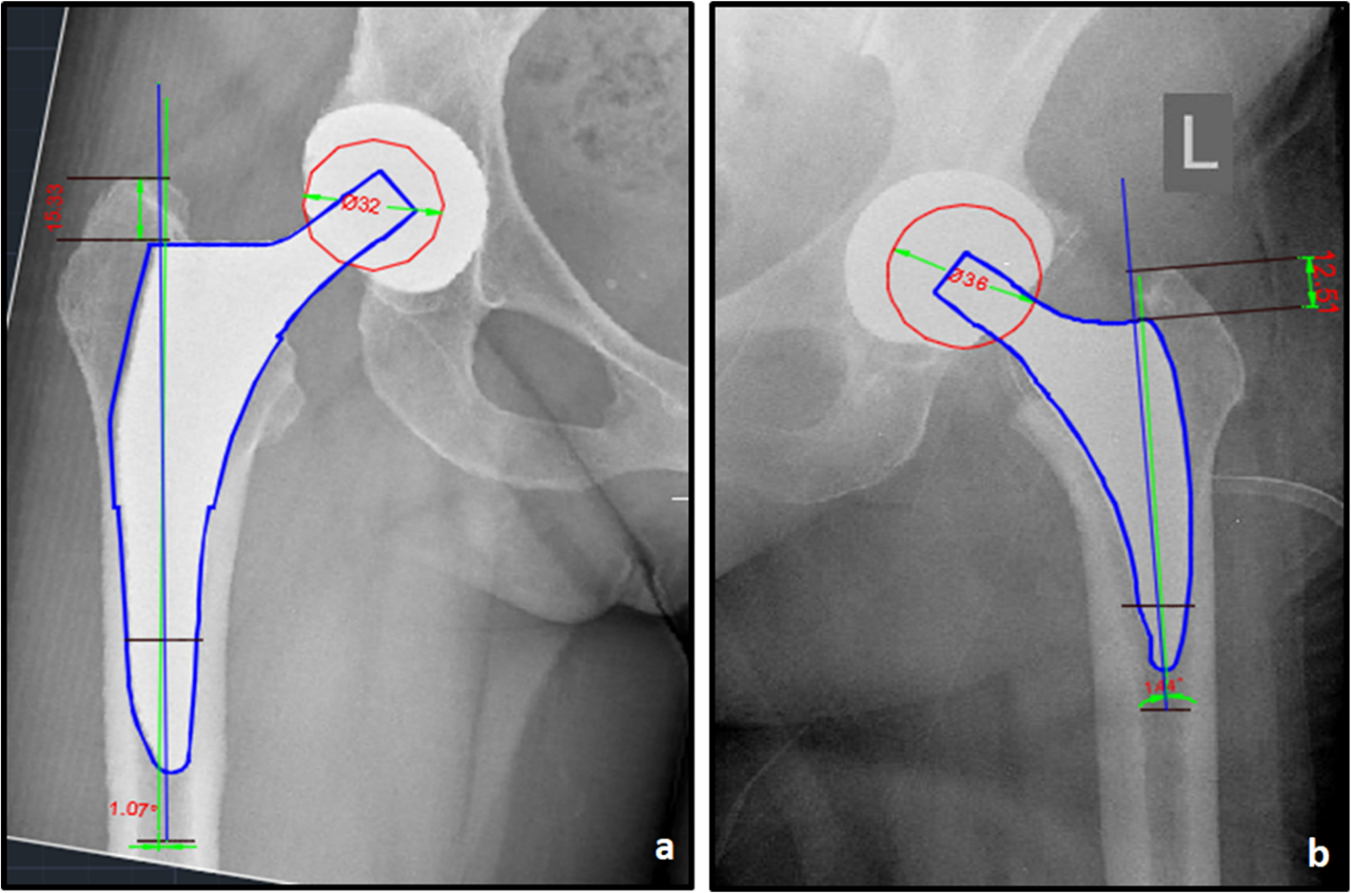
AutoCAD template image on the AP pelvis radiograph showing subsidence calculation and the measurements of stem coronal alignment (angle between the longitudinal axis of the stem—green line, indicated on templates provided by the manufacturer, relative to the axis of the proximal femoral shaft—blue line). Radiographs were calibrated based on the known size of the femoral head. a Tri-Lock BPS. b Minima S
